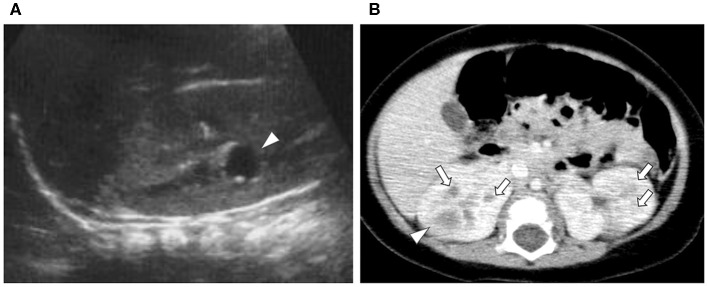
Ultrasound and contrast-enhanced computed tomography (CT). (A) Ultrasound at first visit (Day 1): a 1-cm low echo cyst-like region in the right kidney (arrowhead). (B) First contrast-enhanced CT (Day 36): the kidney is enlarged. Poorly enhanced masses with enhanced borders were found in both kidneys (arrowhead). Two to three patchy poorly contrasted areas were found in both kidneys, but it was difficult to identify if they were cysts or infectious lesions (arrow).
